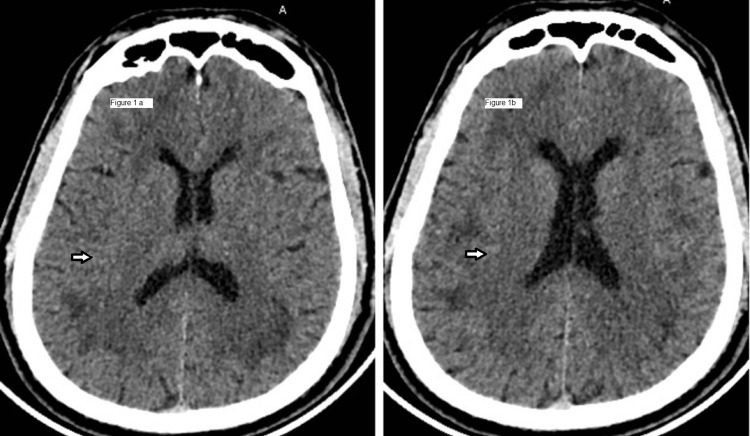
Non-contrast computed tomography (NCCT) head showing bilateral patchy subcortical hypodensities with patchy areas of loss of gray-white matter differentiation in cerebral hemisphere suggestive of white matter edema. Figure 1a and Figure 1b are different cut sections of NCCT head, both showing bilateral patchy hypodensities with patchy areas of loss of gray-white matter differentiation in cerebral hemisphere.
